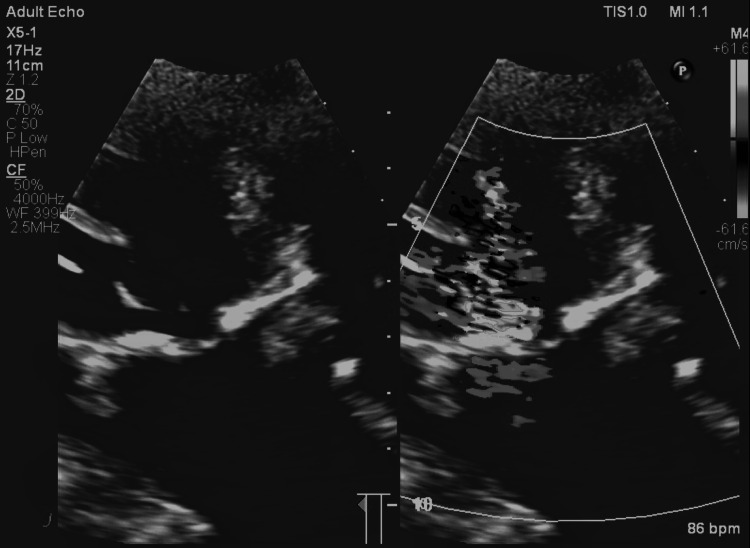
Color Doppler echocardiography demonstrating turbulent systolic flow across the aortic valve
